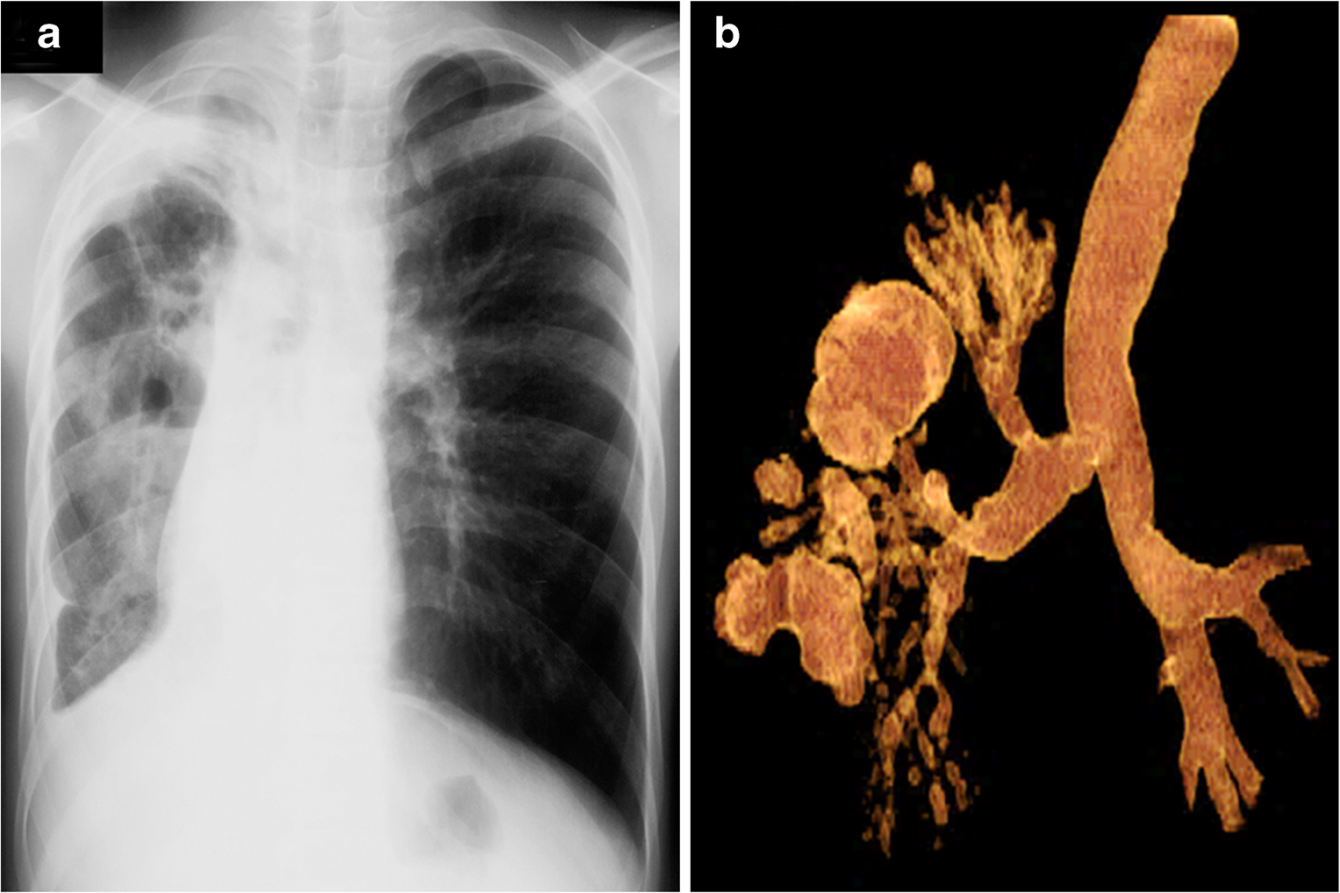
Post-primary tuberculosis in a 14-year-old girl. a, b Posteroanterior chest radiograph (a) and volume-rendered CT (b) show cavitations, traction and cystic bronchiectasis in the right lung
